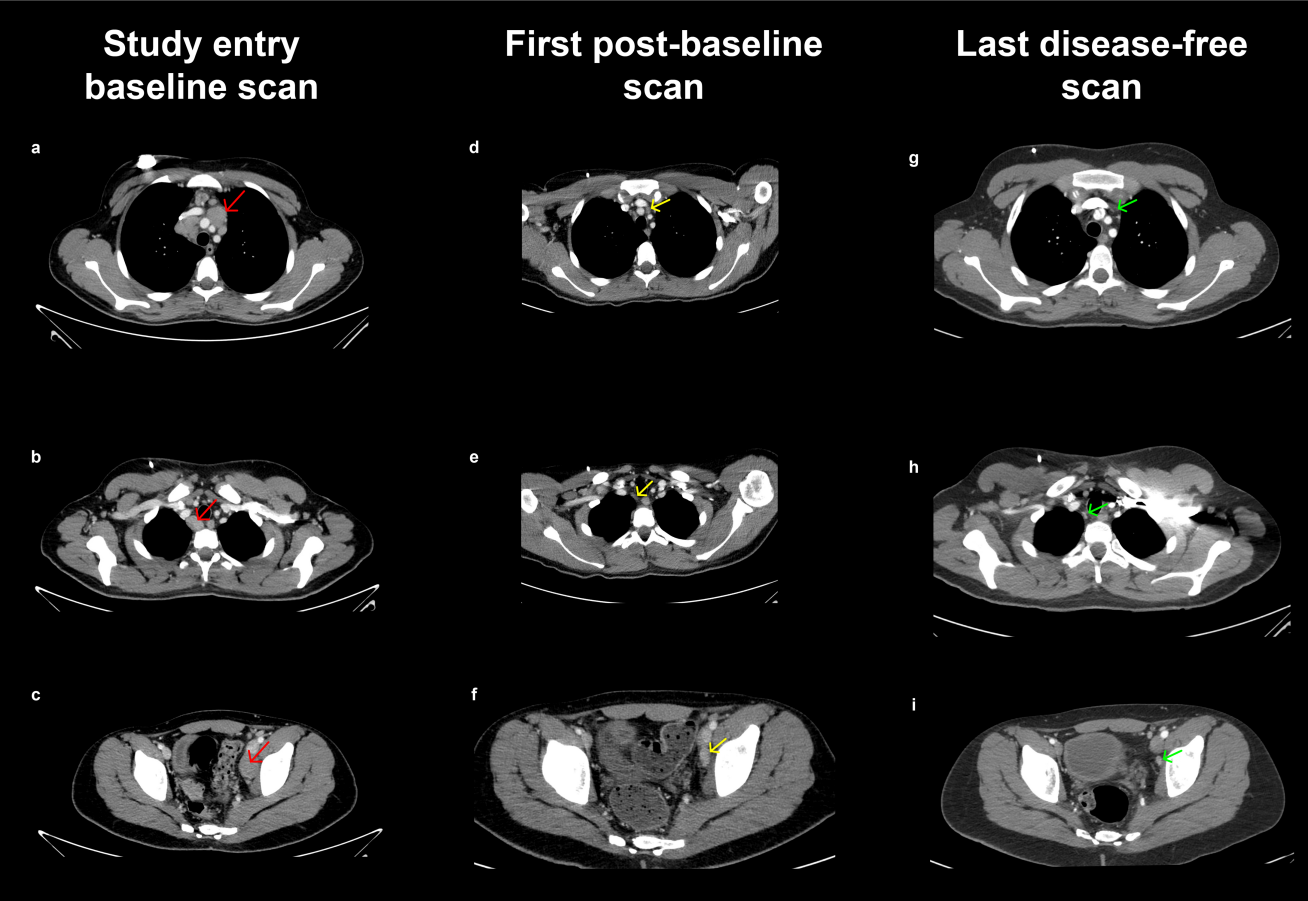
Tumors at JPCS study entry, first post-baseline scan, and last disease-free scan. (a–c) CT scan of the chest, abdomen, and pelvis with intravenous contrast at baseline. Multiple tumor lymphadenopathies were identified: bilateral supraclavicular, mediastinal, and an adenopathic conglomerate in the left iliac axis. (d–f) First post-baseline CT scan of the chest, abdomen, and pelvis with intravenous contrast. A significant reduction in the size and number of bilateral supraclavicular, mediastinal, retroperitoneal, and left iliac axis adenopathy is observed compared to the previous scan. (g–i) Last disease-free CT scan of the chest, abdomen, and pelvis with intravenous contrast showing complete resolution of tumor lymphadenopathy initially identified as target lesions. CT, computed tomography.
